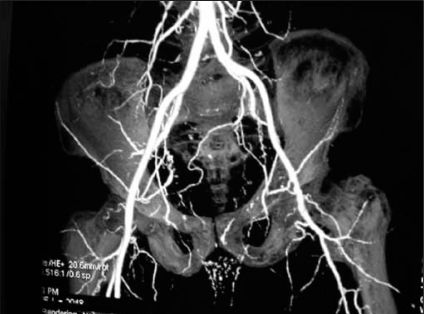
CT angiogram showing multiple areas of stenosis and occlusion in branches of internal iliac arteris bilaterally
